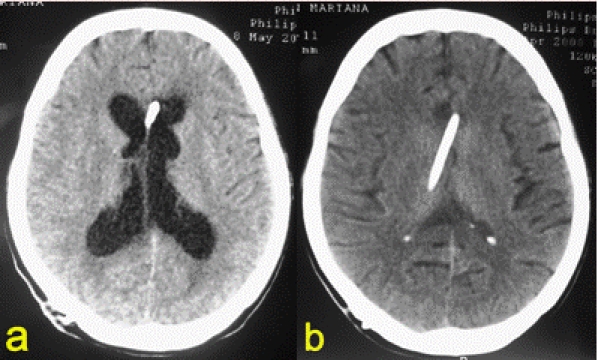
Cerebral CT. a. preoperative; b. postoperative
